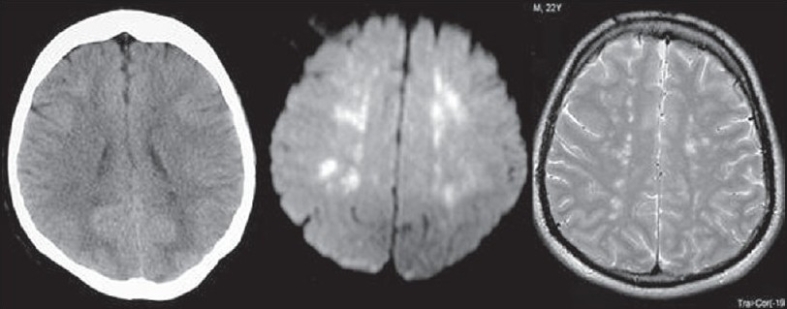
CT image showing minimal hypodense changes in periventricular region, which are more evident in DWI and T2WI as areas of high signals. Constellation of findings along with clinical data is characteristic for FES.
